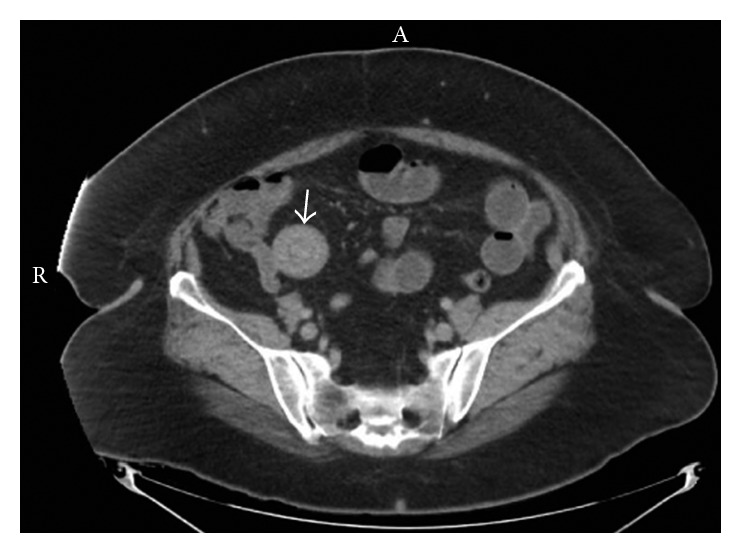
Axial abdominal computed tomography revealing ileoileal intussusception (arrow).
